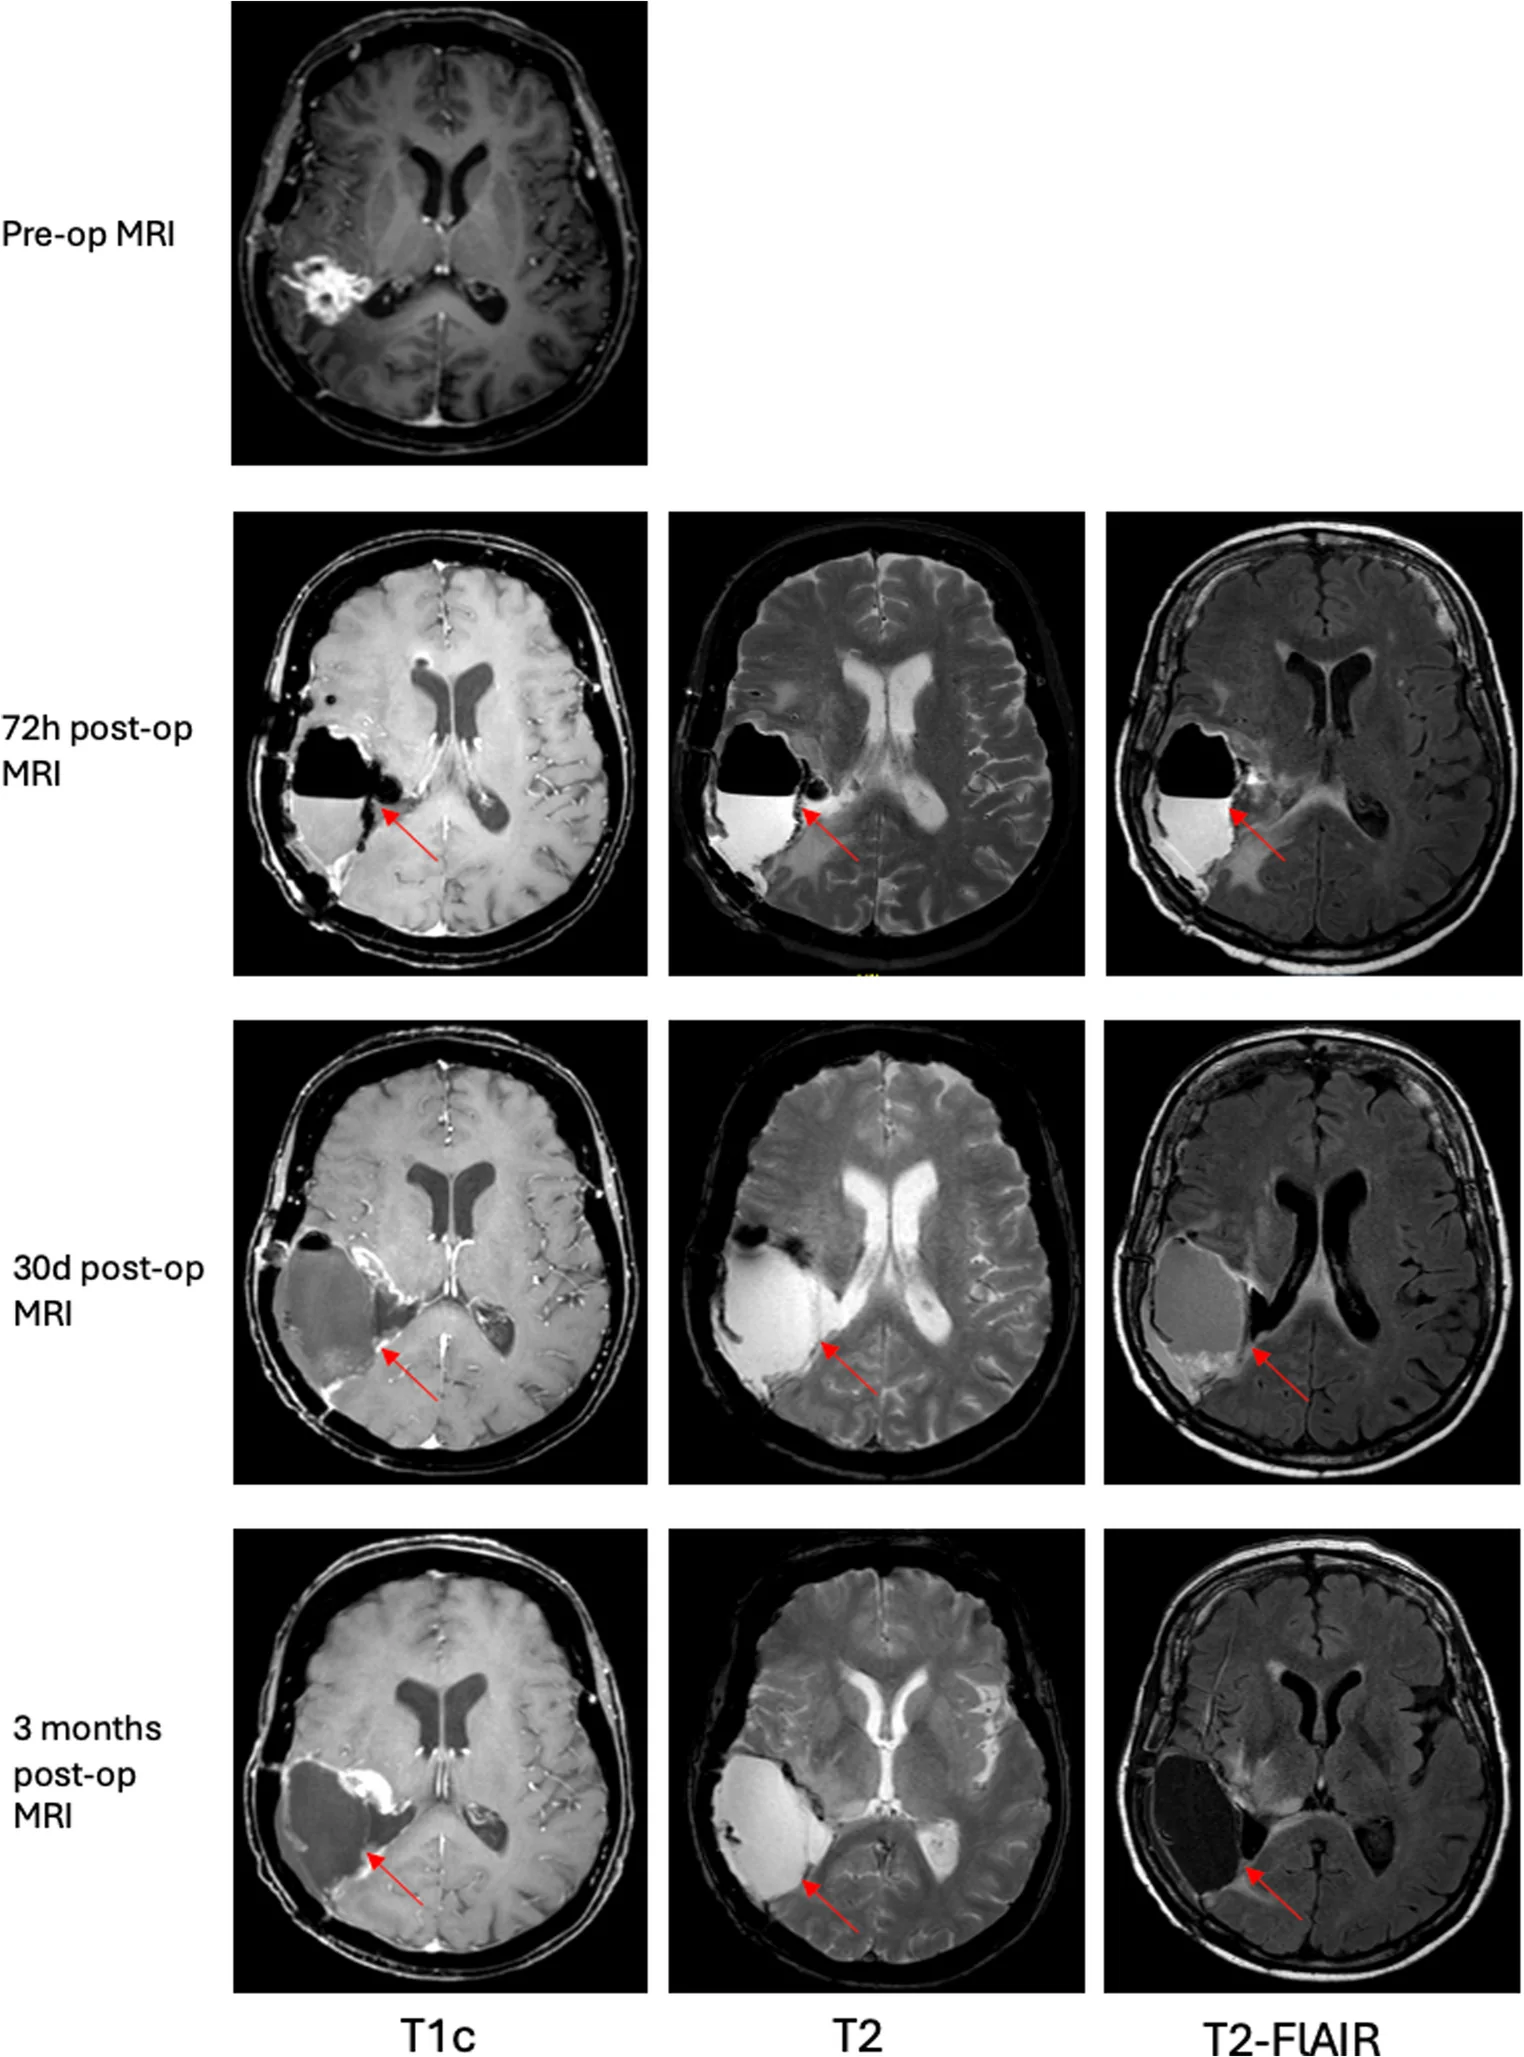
Representative axial MRI of a patient undergoing resection of recurrent glioblastoma with intraoperative ventricular entry and repair of the ependymal defect using TachoSil® (red arrows). The top row shows the preoperative contrast-enhanced T1-weighted (T1c) image, demonstrating an enhancing recurrent right temporoparietal glioblastoma abutting the lateral ventricular system. Postoperative MRI obtained at 72 h, 30 days, and 3 months is shown in the subsequent columns, with T1c, T2-weighted, and T2-FLAIR sequences displayed for each time point. Red arrows indicate the ventricular repair site, which appears as a thin linear interface separating the resection cavity from the ventricular lumen and remains identifiable throughout follow-up, consistent with durable ventricular sealing. No evident imaging features suggestive of transependymal CSF leakage are observed during follow-up
